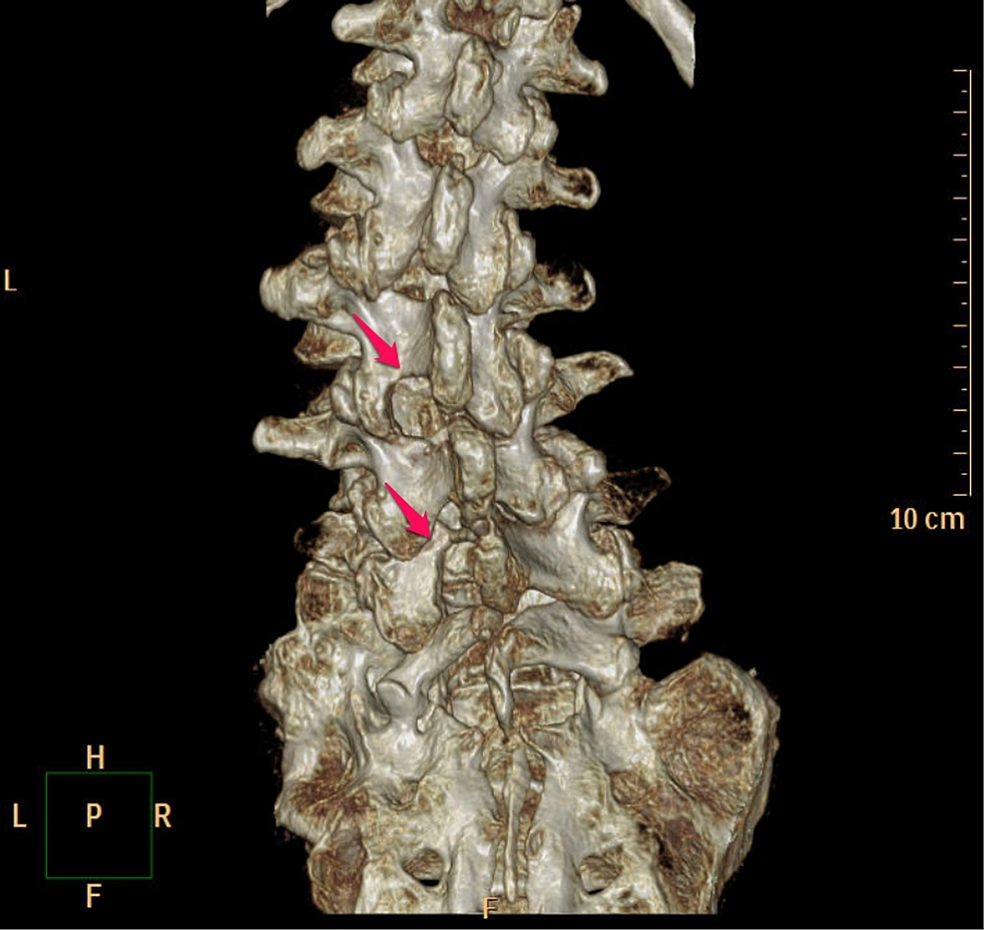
3D-CT after decompression surgery. Decompression was performed at the left L3/4 and L5/6 vertebral levels. 3D-CT: three-dimensional computed tomography
